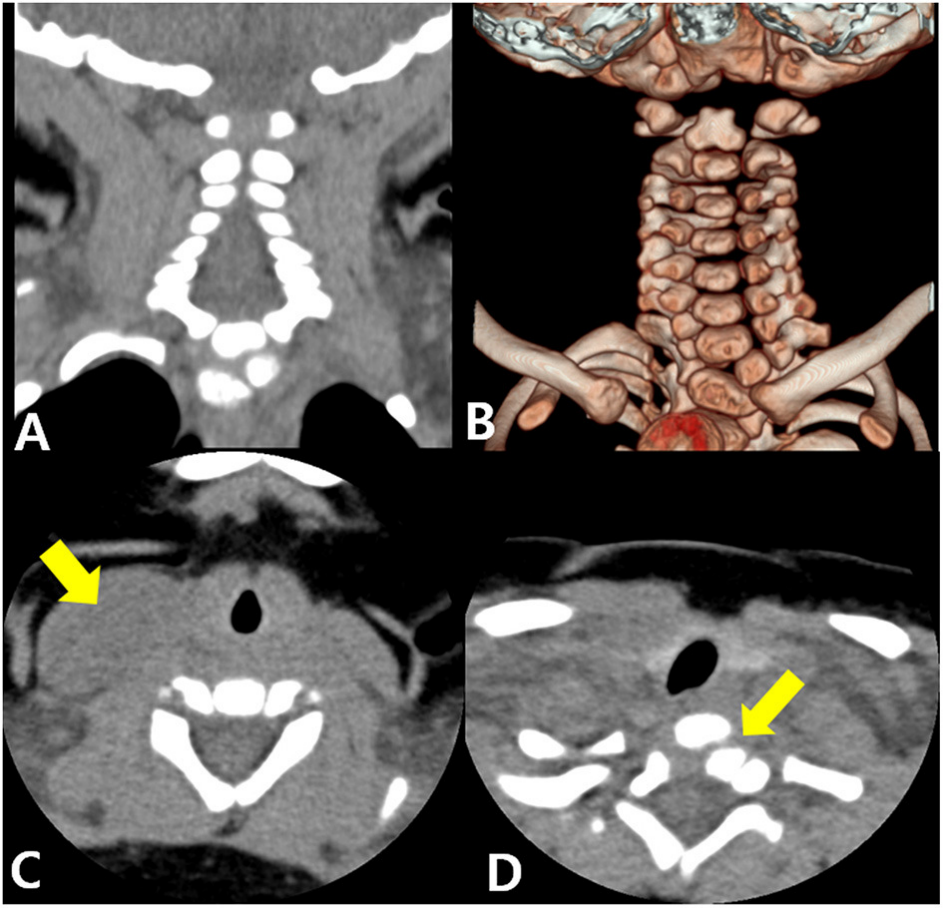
Three-dimensional volume-rendered computed tomography. Coronal image (A) and 3-dimensional image (B) showing T1 butterfly vertebra. Axial cervical image showing enlargement of the sternocleidomastoid muscle (arrow) at the C7 level (C) and a hemivertebra (arrow) at the T1 level (D).
